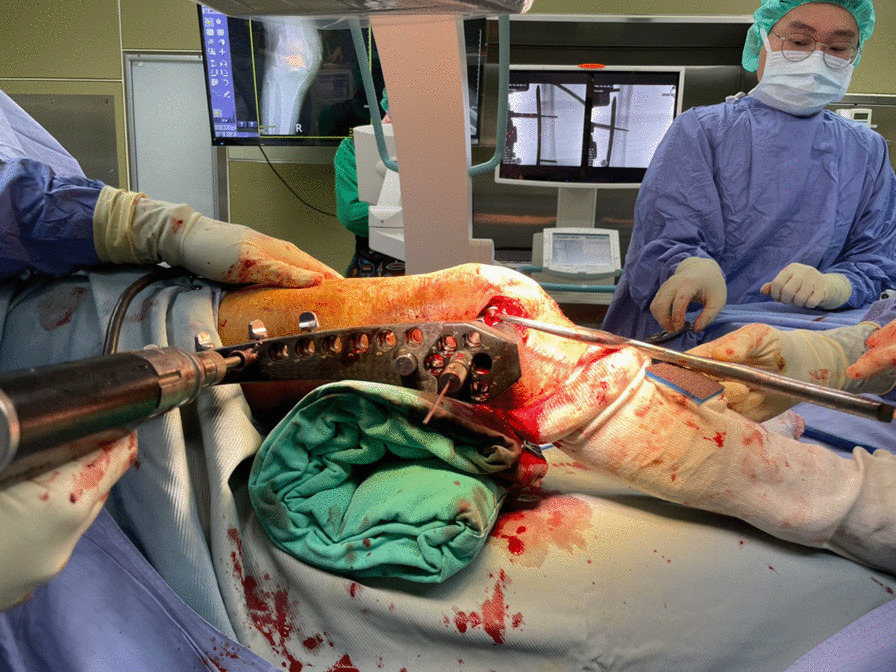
Proximal screws applied through puncture windows, each approximately 1 cm, under the guidance of intraoperative radiography
